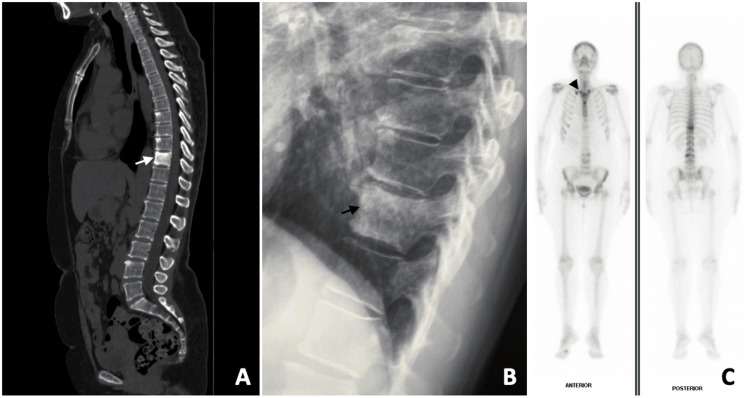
Sagittal view of a CT scan showing an ivory vertebra at T10 (white arrow) and multiple hyperdense lesions in the thoracic and lumbar spine (A). Lateral radiography of the thoracic spine showing the aspect of an ivory vertebra on T10 (black arrow) (B). Anterior and posterior views of whole-body bone scintigraphy with 99mTc-methylene diphosphonate. Increased tracer uptake was observed in both sternoclavicular joints and the sternum (“bull’s head” sign) (black arrowhead), as well as in the upper thoracic and lumbar vertebrae (C).
